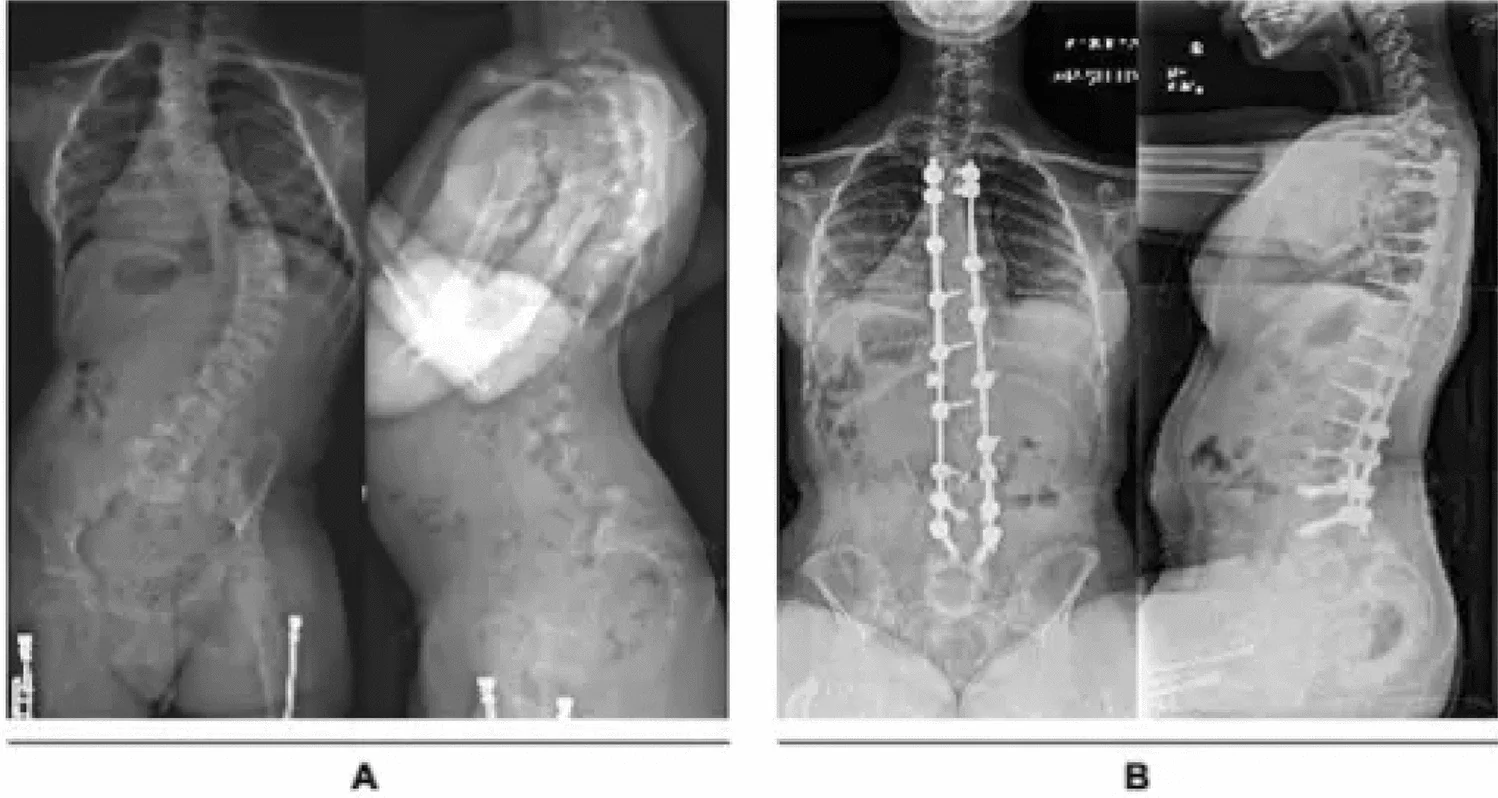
A Preoperative radiographs of a 15-year-old female with type III osteogenesis imperfecta (OI) showing curvatures of 75° and 55°. Preoperative measurements included apical vertebral translation (AVT) of 77 mm, thoracic kyphosis of 22°, thoracolumbar kyphosis of 33°, and lumbar lordosis of 75°. Preoperative SRS-22 total score was 3.2 (pain 4.0, function 3.0, self-image 2.2, mental health 3.4, satisfaction 4.0), CP CHILD total score was 60 (activities of daily living 100, positioning/transferring/mobility 69, comfort and emotions 57.5, communication and social interaction 65, health 60), and PROMIS T-scores were peer relationships 10, fatigue 15, pain interference 18, and physical function mobility 18. B 2-year postoperative radiographs following posterior spinal fusion from T3 to L4 with segmental pedicle screw instrumentation and cement augmentation at the proximal (T3, T4) and distal (L2, L3, L4) foundation levels. Radiographic measurements showed correction of major curves to 11° and 12° with AVT of 14 mm. Sagittal parameters were corrected to thoracic kyphosis of 30°, thoracolumbar kyphosis of 22°, and lumbar lordosis of 57°. Patient-reported outcomes improved with SRS-22 total score of 4.1 (pain 4.2, function 4.0, self-image 4.4, mental health 4.0, satisfaction 4.0), CP CHILD total score of 65 (activities of daily living 100, positioning/transferring/mobility 69, comfort and emotions 87, communication and social interaction 68, health 90), and PROMIS T-scores of peer relationships 14, fatigue 9, pain interference 13, and physical function mobility 14
